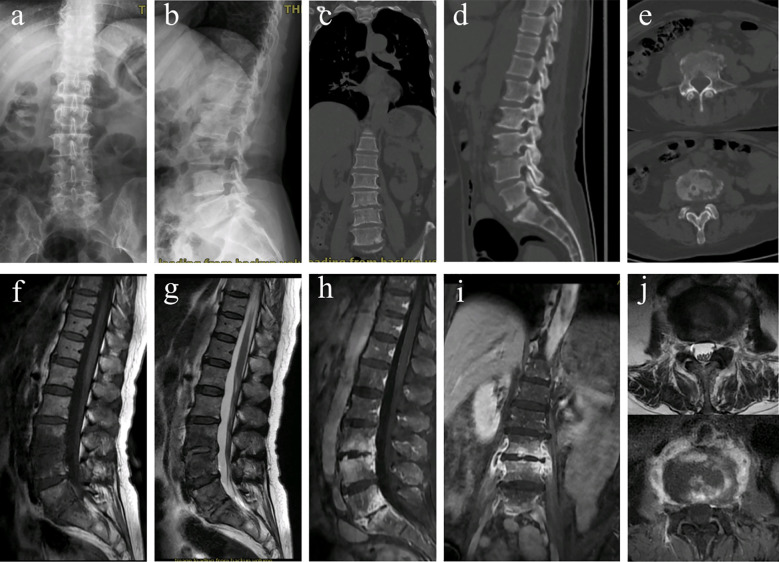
Representative pre-procedural imaging of a patient with lumbar brucellar spondylodiscitis (BS). (a, b) Preoperative anteroposterior and lateral lumbar radiographs. (c–e) Preoperative computed tomography (CT) scans showing osseous destruction of the opposing vertebral endplates at the L3–4 and L5–S1 levels. (f–j) Preoperative T2-weighted magnetic resonance imaging (MRI) demonstrating abnormally increased signal intensity within the intervertebral discs at L3–4 and L5–S1, accompanied by bone marrow edema in the adjacent vertebral bodies, which are typical imaging manifestations of BS.
